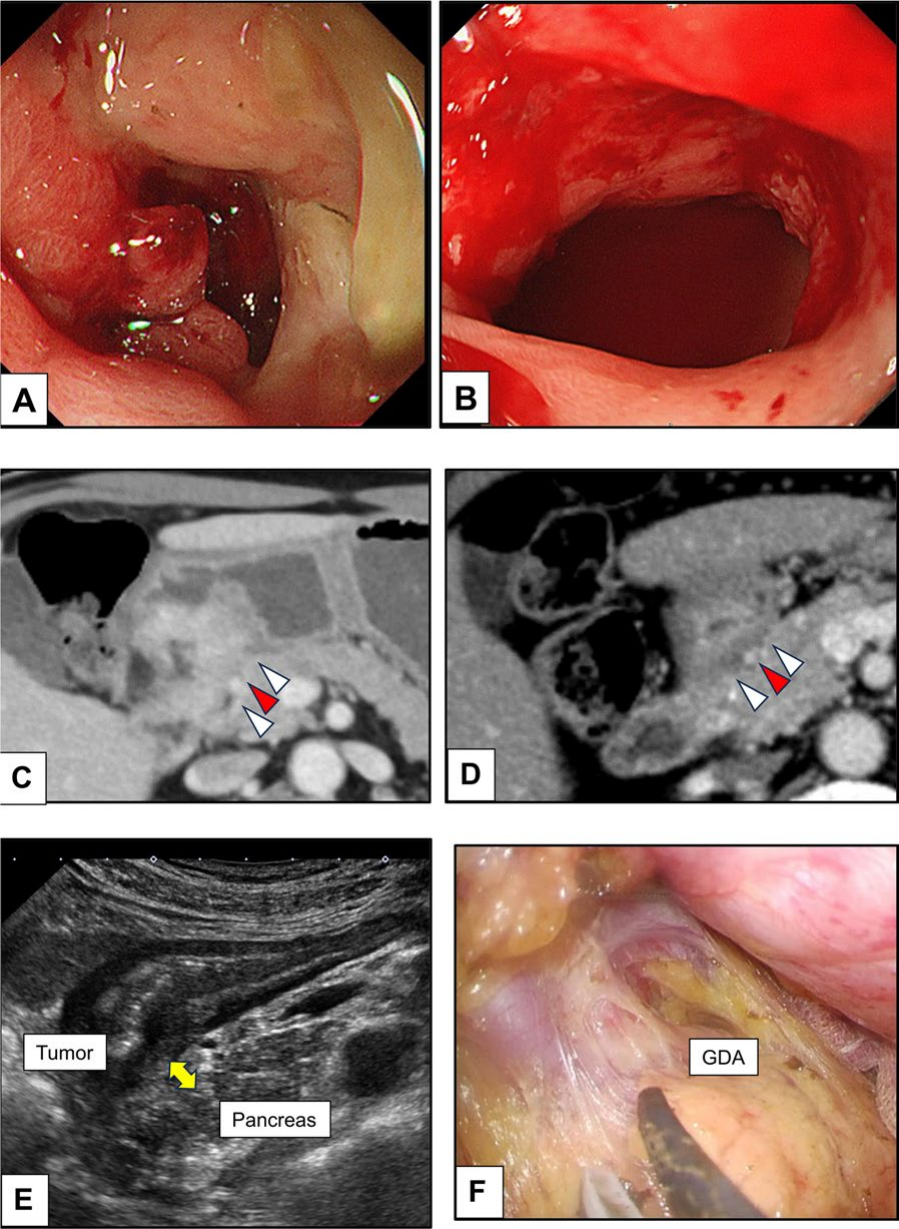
Findings of Case 1. A Gastric cancer extending from the pylorus to the duodenal bulb. B Tumor has shrunk after four courses of perioperative chemotherapy. C Tumor is in close contact with the pancreas (white arrowhead) and the gastroduodenal artery (GDA) (red arrowhead). D Area between the tumor and the pancreas contains a single layer of low-density area after perioperative chemotherapy. E Abdominal ultrasound indicating the presence of a space between the tumor and pancreas (yellow arrow). F Area between the tumor and the pancreas shows only edematous changes
